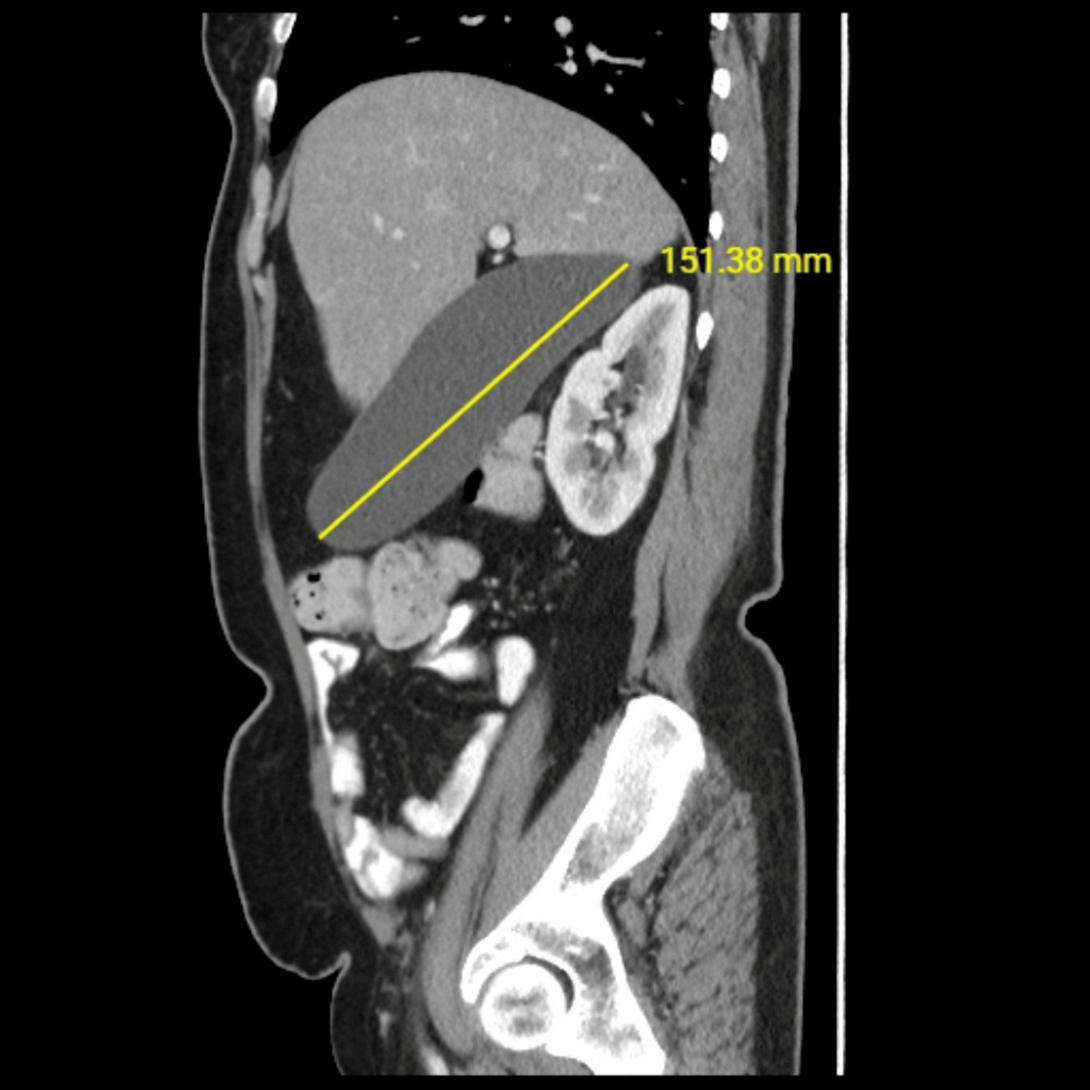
Abdominal CT at the first presentation Abdominal CT from the first presentation (three years before the cholecystectomy detailed in this case report) showing a distended gallbladder.
